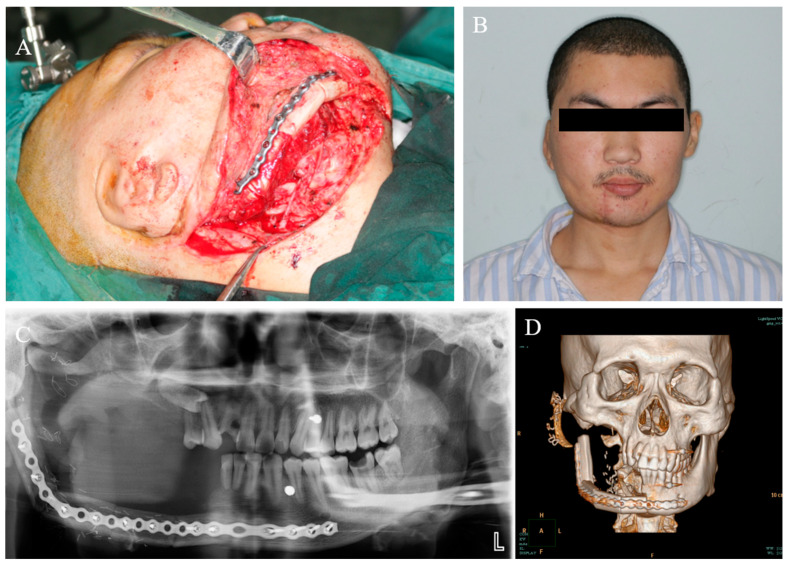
The patient received mandibular defect reconstruction with vascularized fibular myocutaneous flap. (A) Surgical photograph. (B) Clinical photograph. (C) Panoramic radiography. (D) Three-dimensional reconstruction.
